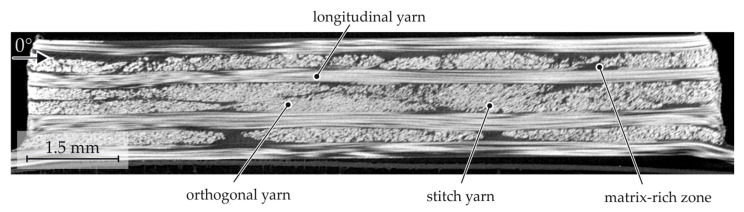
CT analysis of the initial material structure of the multilayered GF-PA6.
